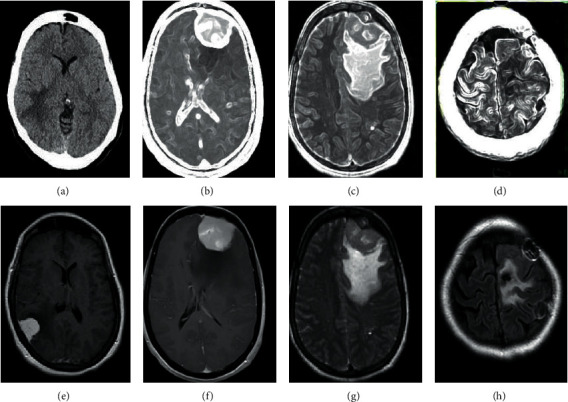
The MRI image of a 48-year-old female patient with the brain tumor. (a–d) FLAIR, T1, T1c, and T2 modal images processed by conventional MRI image algorithms, respectively. (e–h) FLAIR, T1, T1c, and T2 modal images processed by CNN algorithm, respectively.
